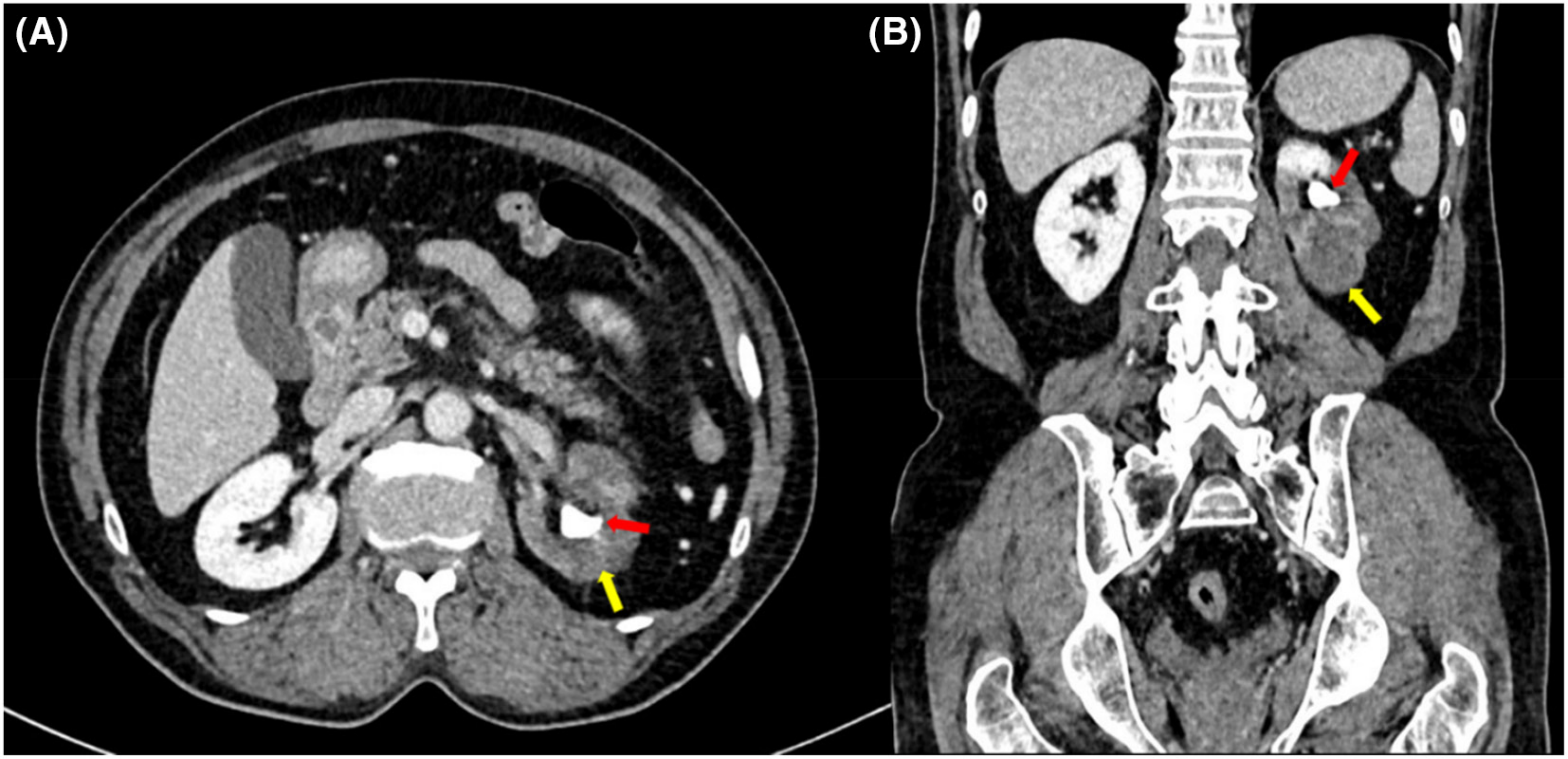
CT abdomen and pelvis with IV contrast (A) Axial and (B) Coronal cuts showing inhomogeneous parenchymal enhancement of left kidney with no excretion (Yellow arrows). A 16 mm calculus is seen in the left renal pelvis (Red arrows).
